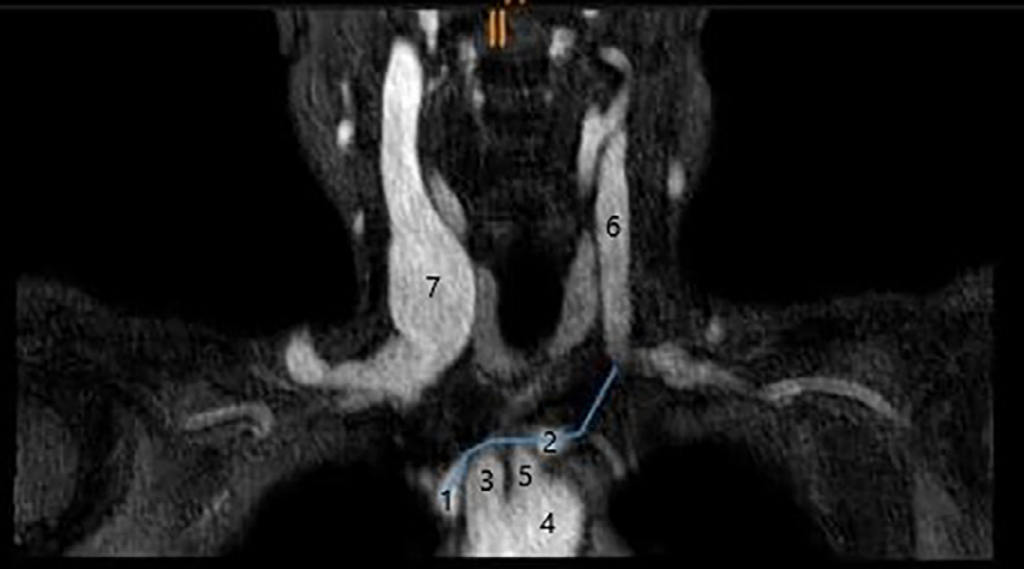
Coronal section: Excessive varicose right internal jugular vein. Contrast neck angio MRI (A,E) Tesla MRI, 4D TRAK Angiography : Vena cava superior; (B) Blue line: Vena brachiocephalica sinistra; (C) Brachiocephalic trunk; (D) Aorta descendence; (E) Arteria carotis communis sinistra; (F) Left internal jugular vein; (G) Right internal jugular vein.
